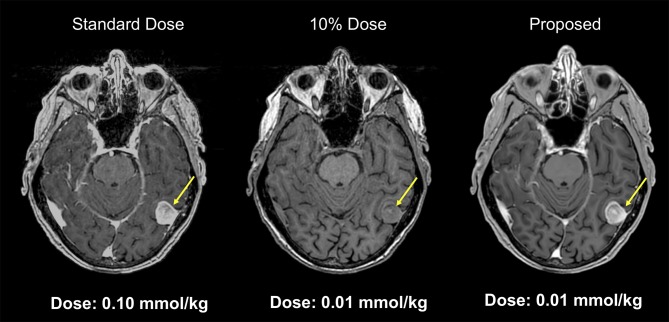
Example of low-dose contrast-enhaced MRI. Results from a deep network for predicting a 100% contrast dose image from a study obtained with 10% of the standard contrast dose. This example MRI is abtained from a patient with menigioma. Such methods may enable diagnostic quality images to be acquired more safely in a wider range of patients (Courtesy of Subtle Medical, Inc.).
